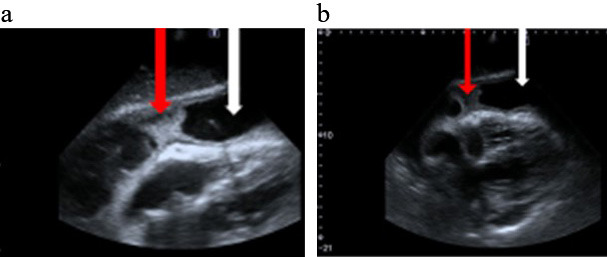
Bedside echocardiography (a,b) Modified sub-sternal
